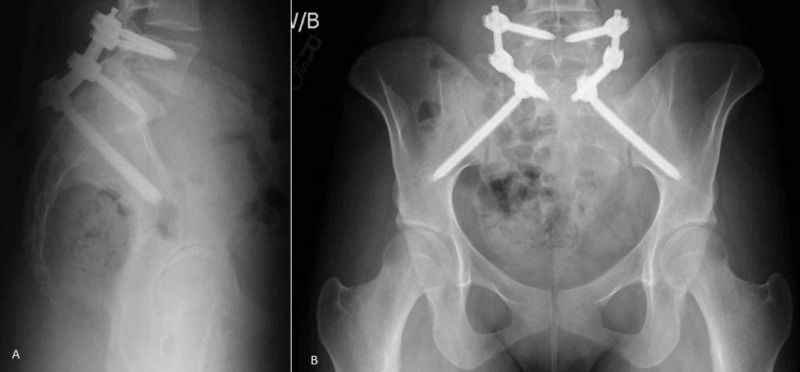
(A) Lateral radiograph of final construct after minimally invasive lumbo-pelvic fixation. Percutaneous fixation was done from L5 to S2. (B) AP radiograph of the minimally invasive lumbo-pelvic fixation. All images are weight bearing
